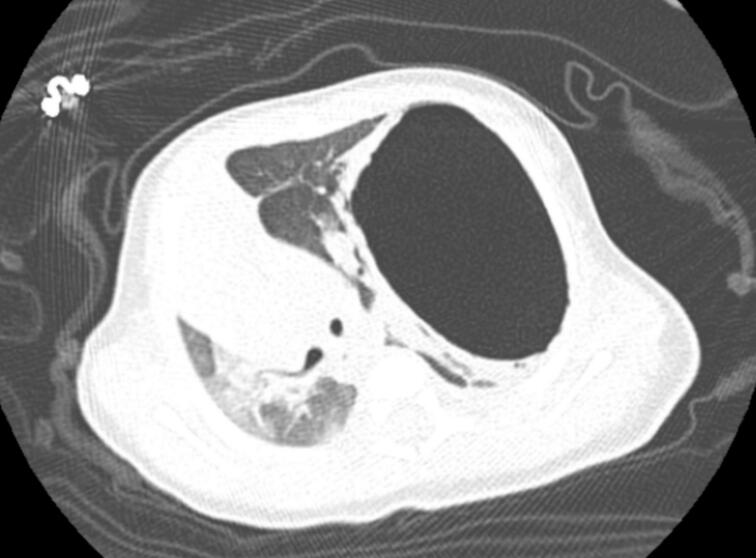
CT scan show there is a large bilocular cystic lesion measuring about 3.5 ∗ 5.5 ∗ (TS ∗ AP), occupying left upper lung lobe associated with surrounding consolidative and ground glass opacities causing a contralateral mediastinal and tracheal shift associated with diffuse bilateral mosaic attenuation along with another small cavitary cystic lesion measuring about 2 mm in diameter. No visible communication with the bronchial system.
